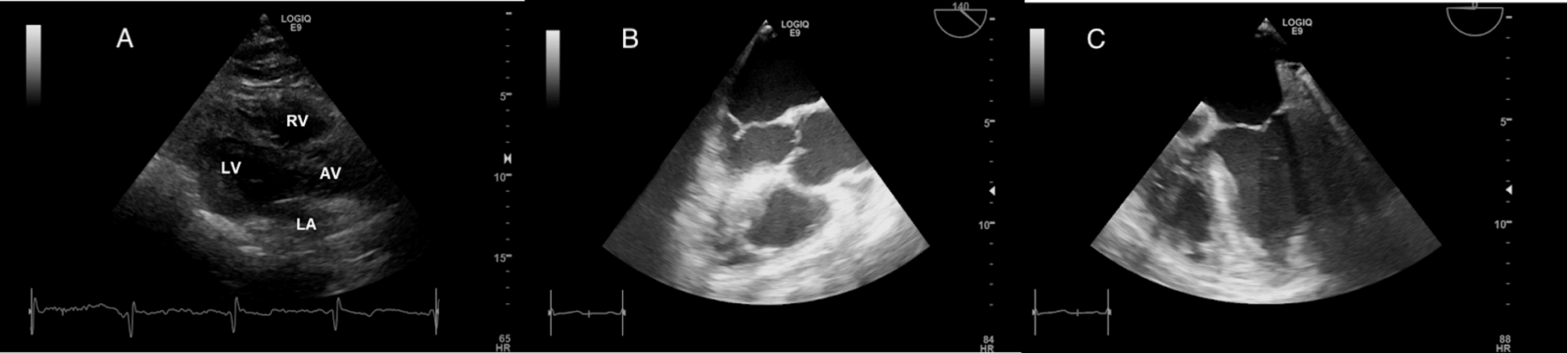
Admission echocardiography images: (A) Four-chamber view from transthoracic echocardiography showing no valve vegetation. (B) Aortic view from transesophageal echocardiography, revealing no vegetation on the aortic valve. (C) Four-chamber view at 0 degrees from transesophageal echocardiography, showing no vegetation on the mitral valve
